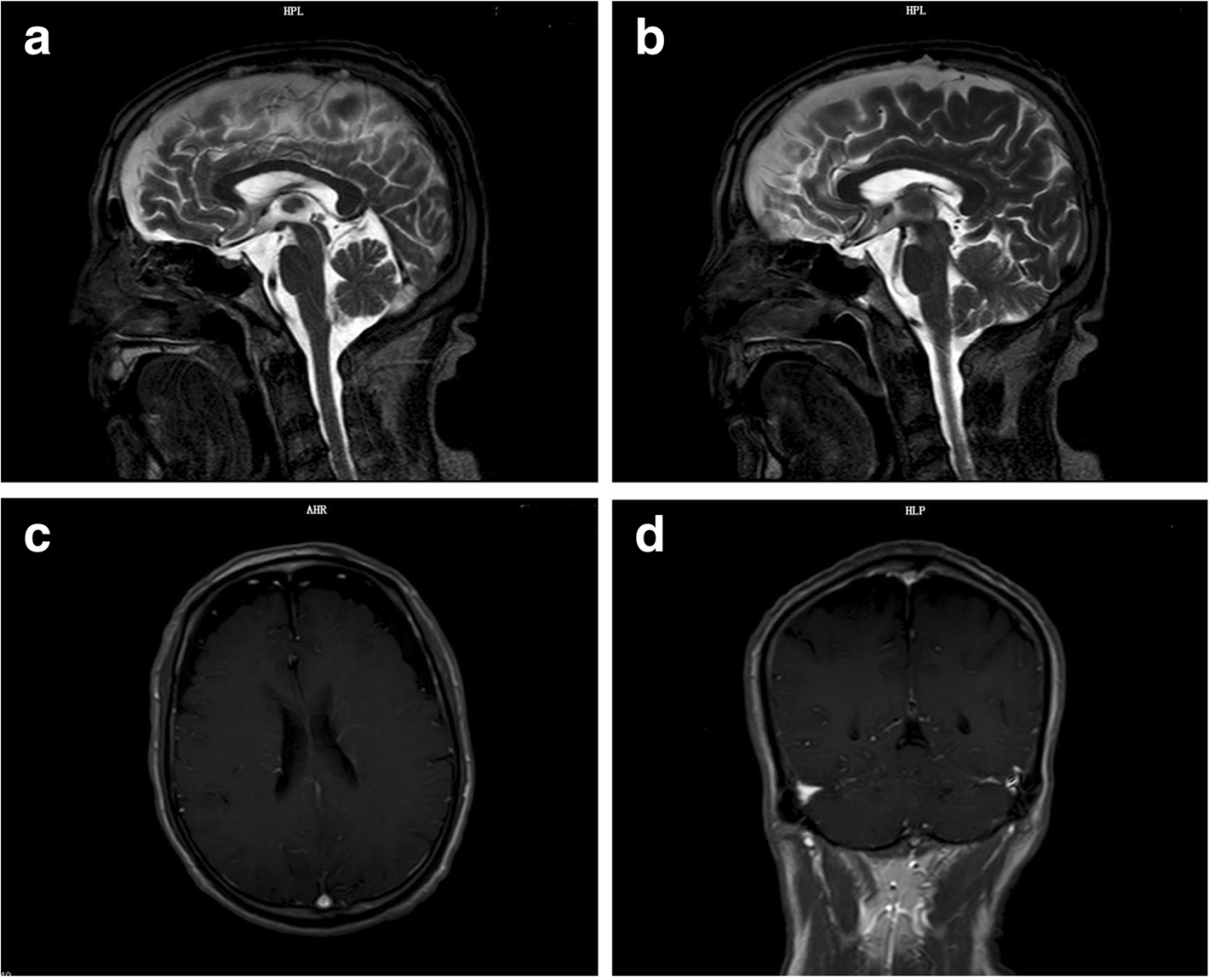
Magnetic resonance imaging (MRI) of the brain. a and b, the T2WI sagittal scan showed no obvious morphological change of the cerebellum; c and d, the enhanced-scanning MRI revealed no sign of brain metastases from bladder cancer
